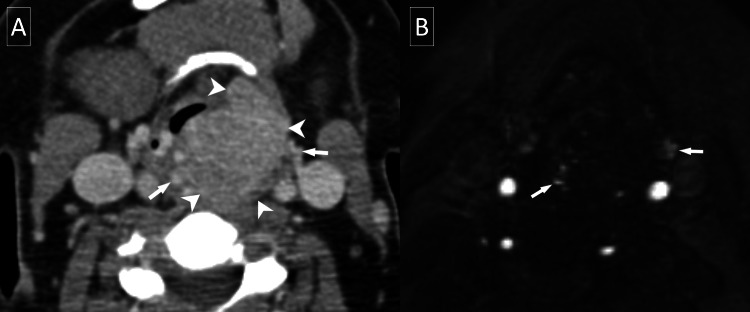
CT of the soft tissue neck with intravenous contrast. Initial axial CT of the soft tissue neck with intravenous contrast (A) demonstrates an enhancing well-circumscribed 4.7 cm mass (arrowheads) centered in the left supraglottic larynx with significant mass effect and no extralaryngeal extension. Extensive surrounding vascularity (arrows) is noted on both the CT and time-of-flight magnetic resonance angiography (B).
